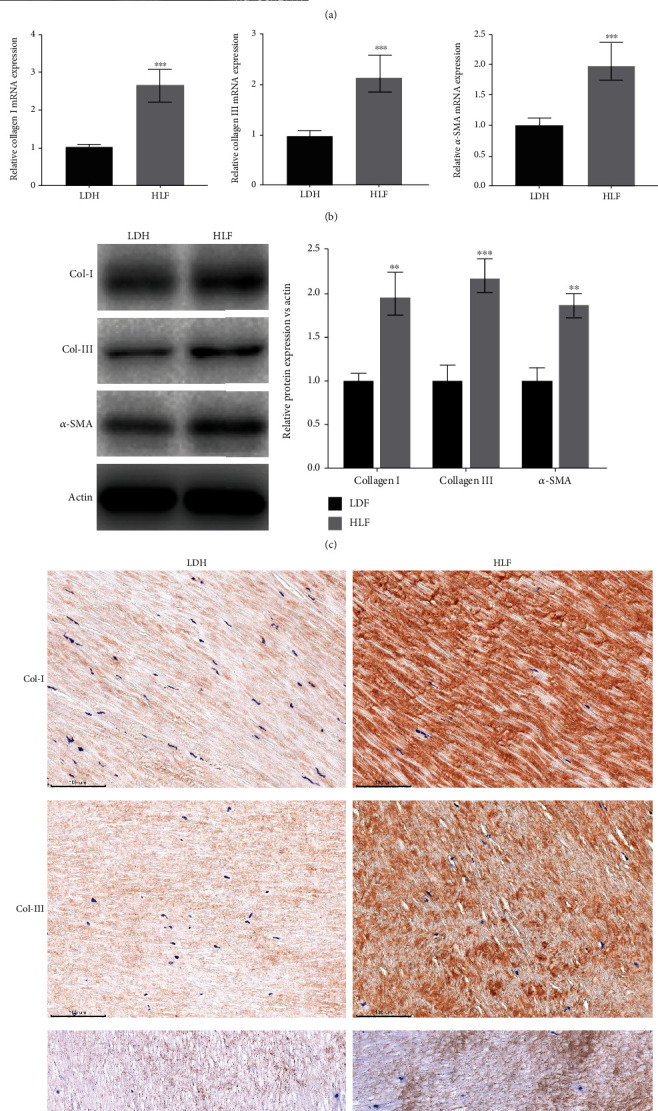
Fibrosis was upregulated in LF tissues from HLF patients. (a) Magnetic resonance imaging (MRI) shows axial views of the lumbar spinal canal in the LDH and HLF patients. (b) Collagen I, Collagen III, and *α*-SMA mRNA levels in the LDH and HLF patients were assessed by qRT-PCR (*n* = 27). ^∗∗∗^*p* < 0.001. (c, d) Collagen I, Collagen III, and *α*-SMA protein expression in the LDH and HLF patients was assessed by western-blot and IHC (*n* = 10). ^∗∗^*p* < 0.01, ^∗∗∗^*p* < 0.001.
